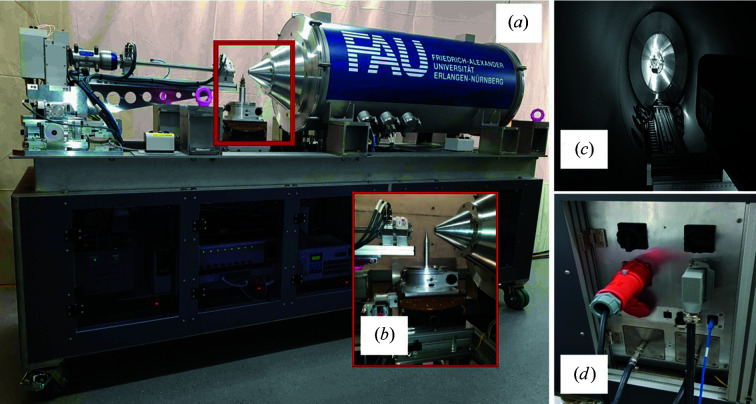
(*a*) Photograph of the portable SAXS instrument for simultaneous SAXS/SANS measurements at D22–ILL. (*b*) Six-axis goniometer for correct sample alignment with respect to both X-ray and neutron beams. (*c*) EIGER R 1M detector with three degrees of freedom to move inside the vacuum chamber. (*d*) The central hub for easy and smooth plug-and-play operation, including power supplies, cooling water and ethernet cable. All system components are enclosed within a single heavy-duty metal non-magnetic chassis.
